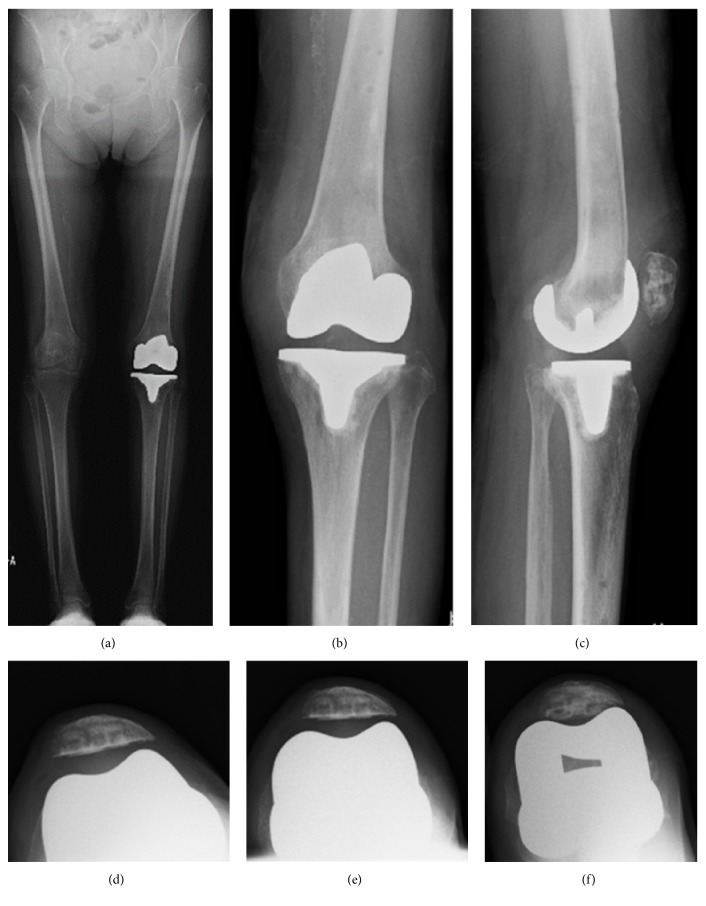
Postoperative plain radiograph. Anteroposterior long leg standing image of both legs (a), anteroposterior one leg standing image of left knee (b), lateral one leg standing image of left knee (c), skyline view of patella at 30 degrees of knee flexion (d), 60 degrees of knee flexion (e), and 90 degrees of knee flexion (f).
